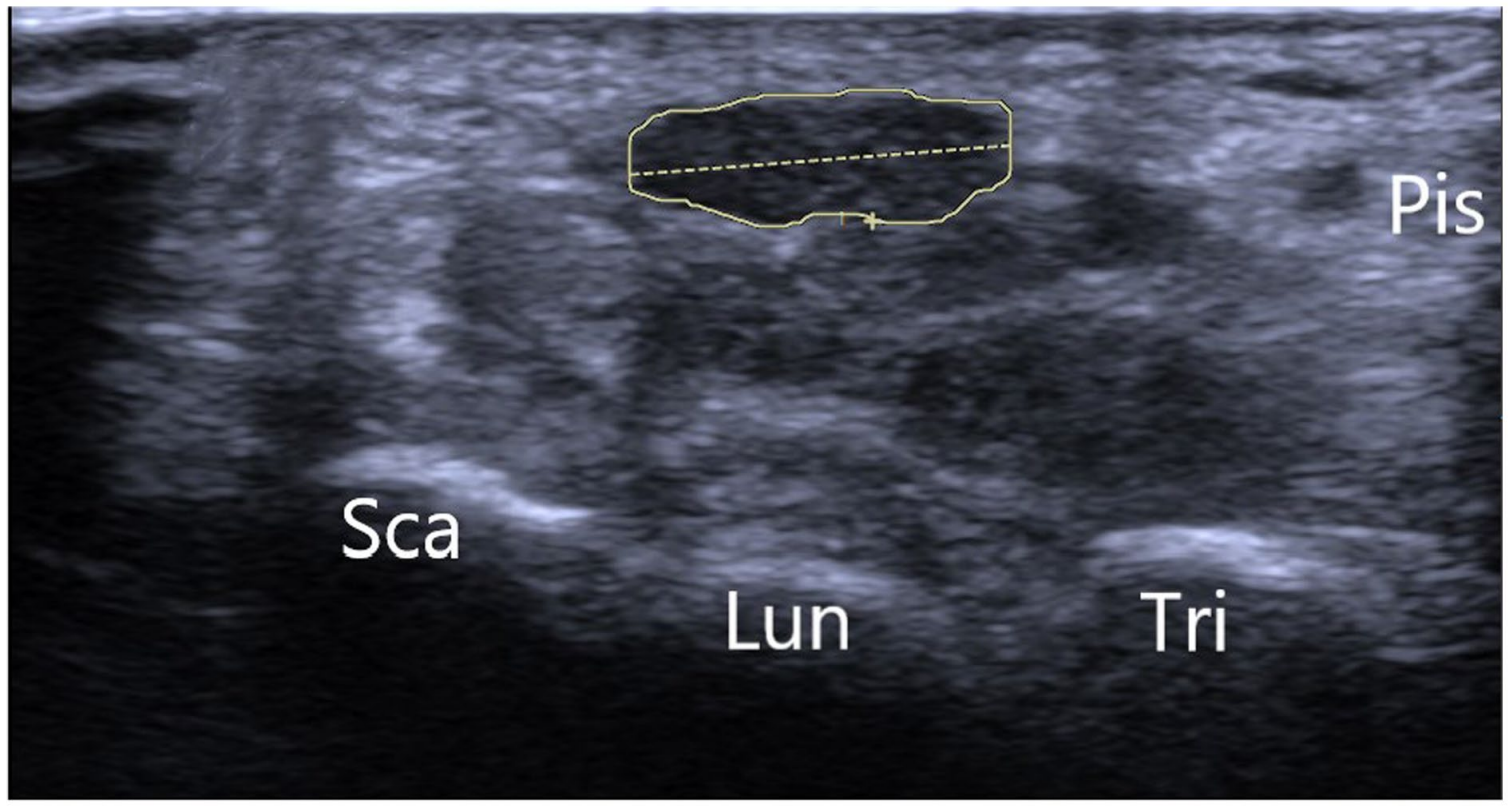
Depicting the B-ultrasonography image of typical carpal tunnel syndrome. *Sca, scaphoid bone; Lun, lunate bone; Tri, triquetral bone; Pis, pisiform bone.
